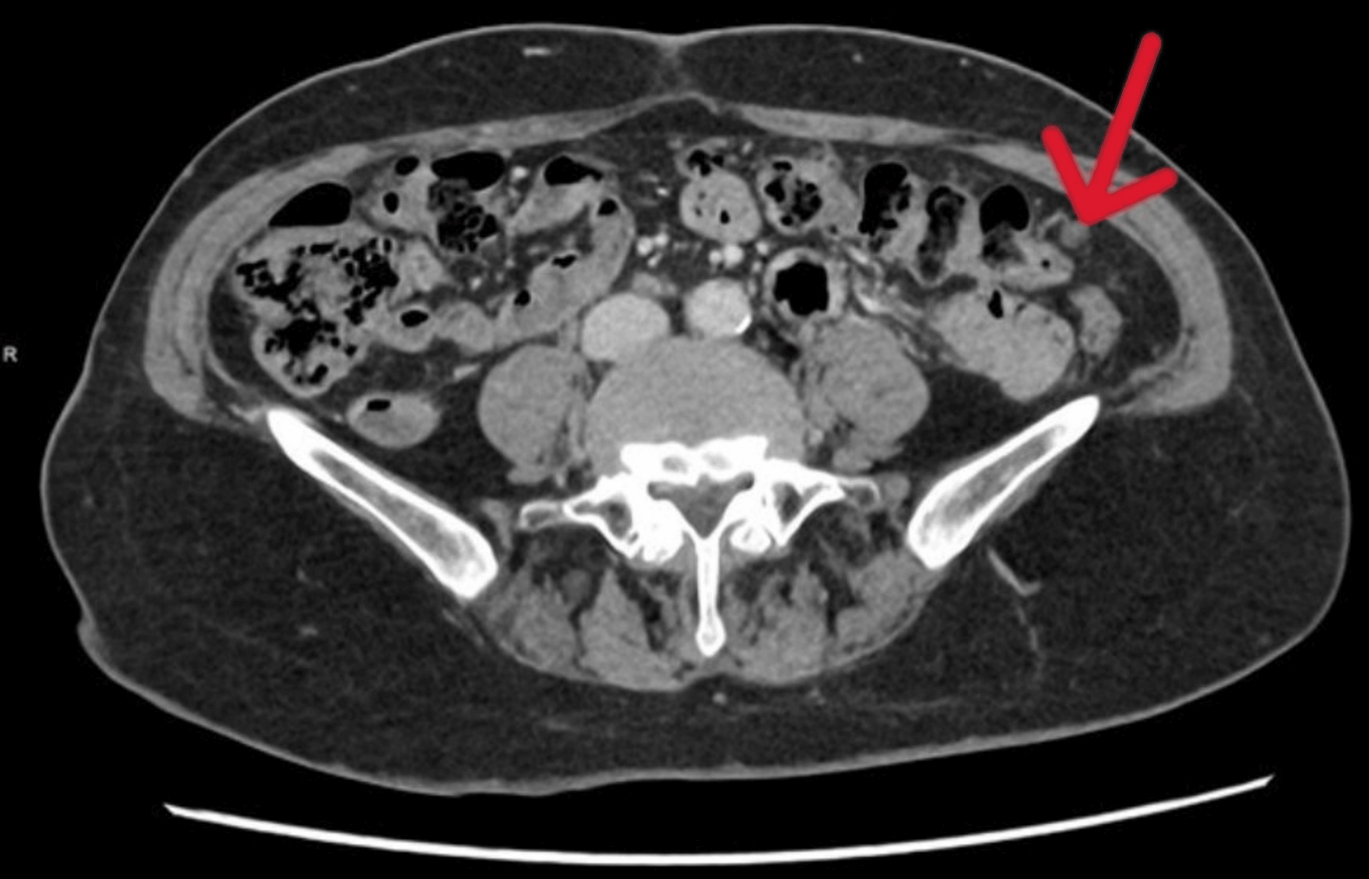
Axial contrast-enhanced CT showing a feeding vessel supplying the peritoneal cyst (arrow).
